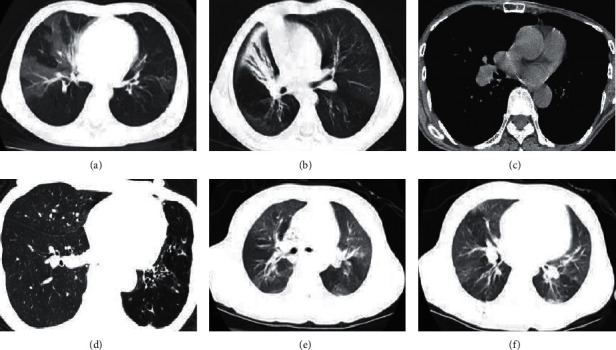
HRCT iterative reconstruction image of BO. (a, b, e, and f) HRCT plain scan images; (c, d) Expiratory phase HRCT images.
